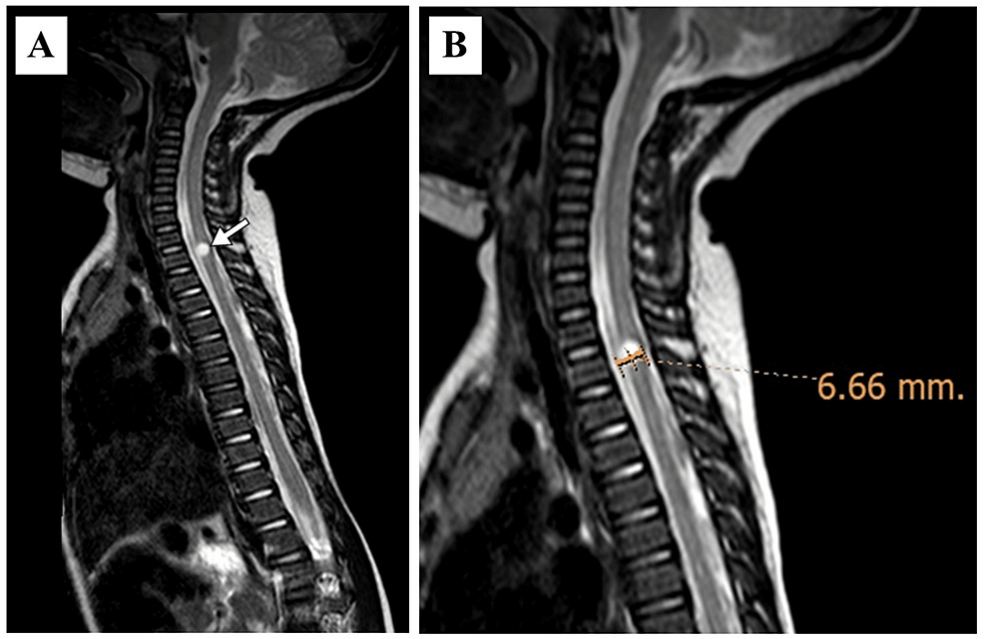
A sagittal T2-weighted magnetic resonance imaging of the spine of a child with Johanson-Blizzard syndrome. (A) A hyperintense cystic lesion at the anterior aspect of the spinal cord at the second thoracic vertebral level (arrow), most likely representing a syringohydromyelia; (B) a close-up image demonstrates the size of the cystic lesion.
